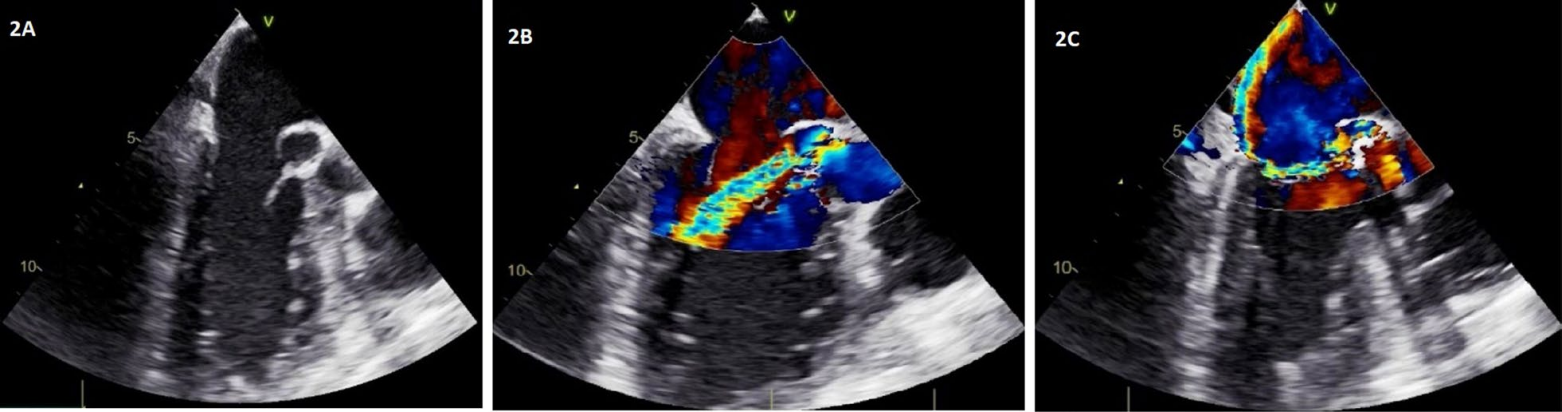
Transesophageal echocardiogram. A Transesophageal echo image showing echo-free space with free communication between the aorta and left atrium, consistent with fistula formation. B Transesophageal echo image with color Doppler, showing flow through fistulous communication. C Transesophageal echo image with color Doppler, showing severe mitral regurgitation with an eccentric posteriorly directed jet. Flow through fistula is also visible
